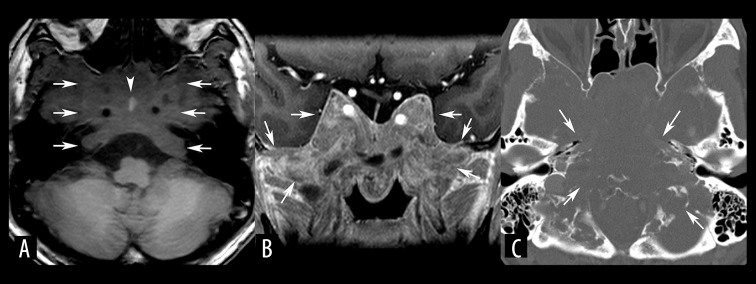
Pituitary adenoma in a 38-year-old man. (A) Axial, non-contrast, T1-weighted MR image shows a large, infiltrating, hypointense mass (arrows) at the central skull base. Bone invasion can be confirmed by the loss of normal high signals from fatty bone marrow. Intratumoral hemorrhage (arrow head) can be also seen. (B) On coronal-reformatted, contrast-enhanced MR image, the mass shows moderate enhancement with a very indistinct margin (arrows). (C) Axial, bone window CT image shows bone destruction of the central skull base (arrows). A pathological analysis revealed a prolactin-producing pituitary adenoma.
